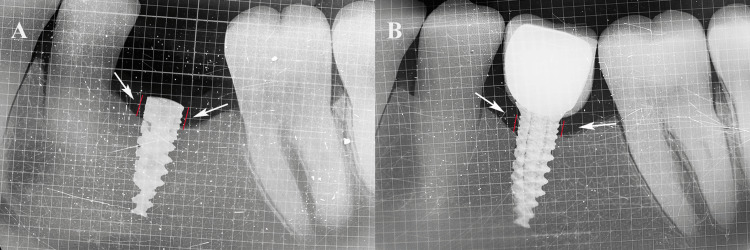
Radiograph findings. Marginal bone loss was measured using 1-mm grid–enabled intraoral periapical radiographs (IOPAs) (A) after three months, at the time of the second surgical procedure, and (B) after six months, post-loading. Red line denotes the distance between the implant shoulder and alveolar crest on mesial and distal sides as marginal bone loss in mm (white arrows). Original IOPAs of the patient from the study were used with the patient's permission.
